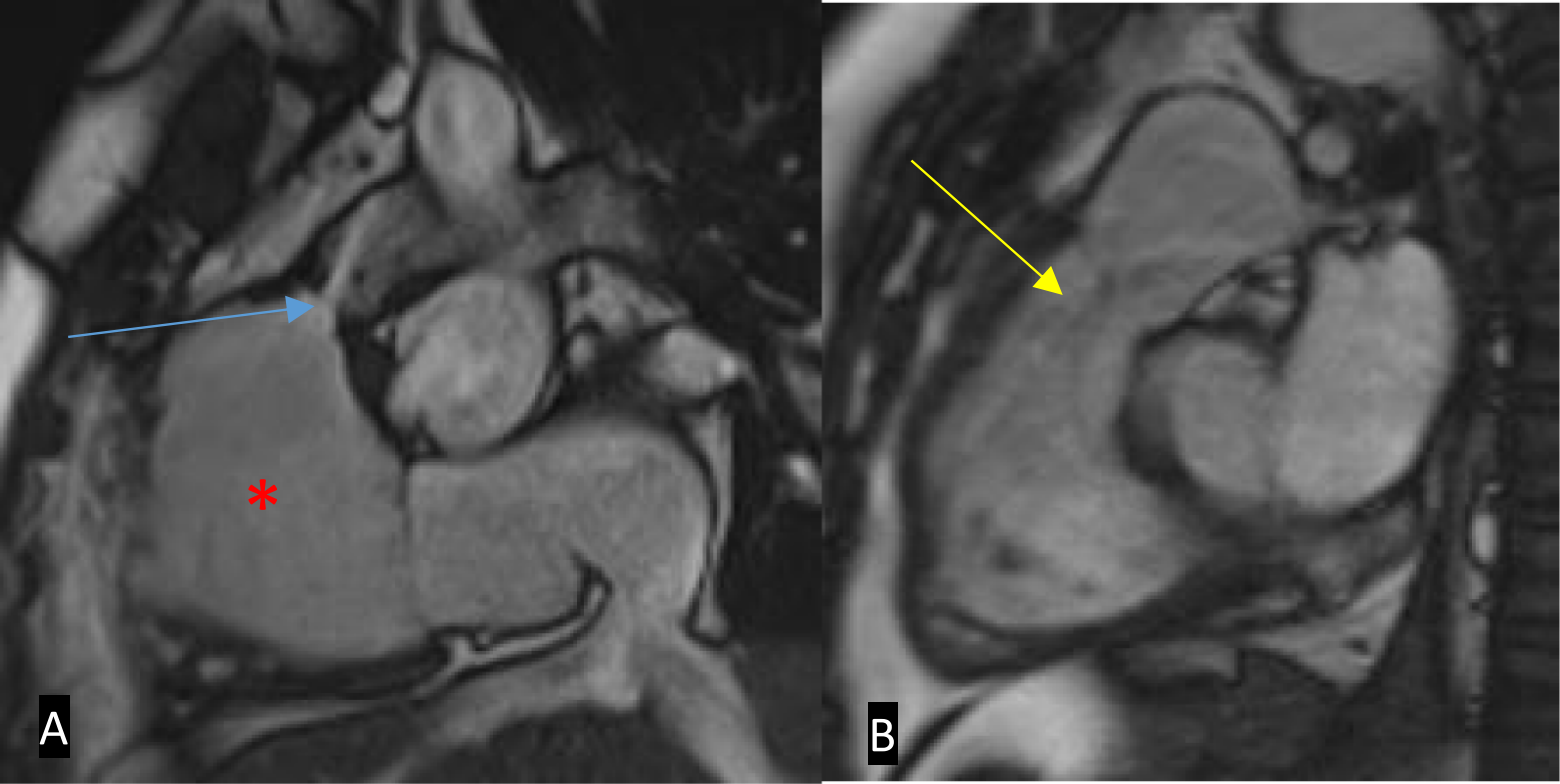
MRI SSFP cine images in two different patients post-TOF repair taken in the RVOT plane. A There is RVOT residual obstruction with a stenotic jet (blue arrow) with RV dilatation (red asterisk). B A jet of pulmonary regurgitation can be seen (yellow arrow). RVOT: right ventricular outflow tract; RV: right ventricle
